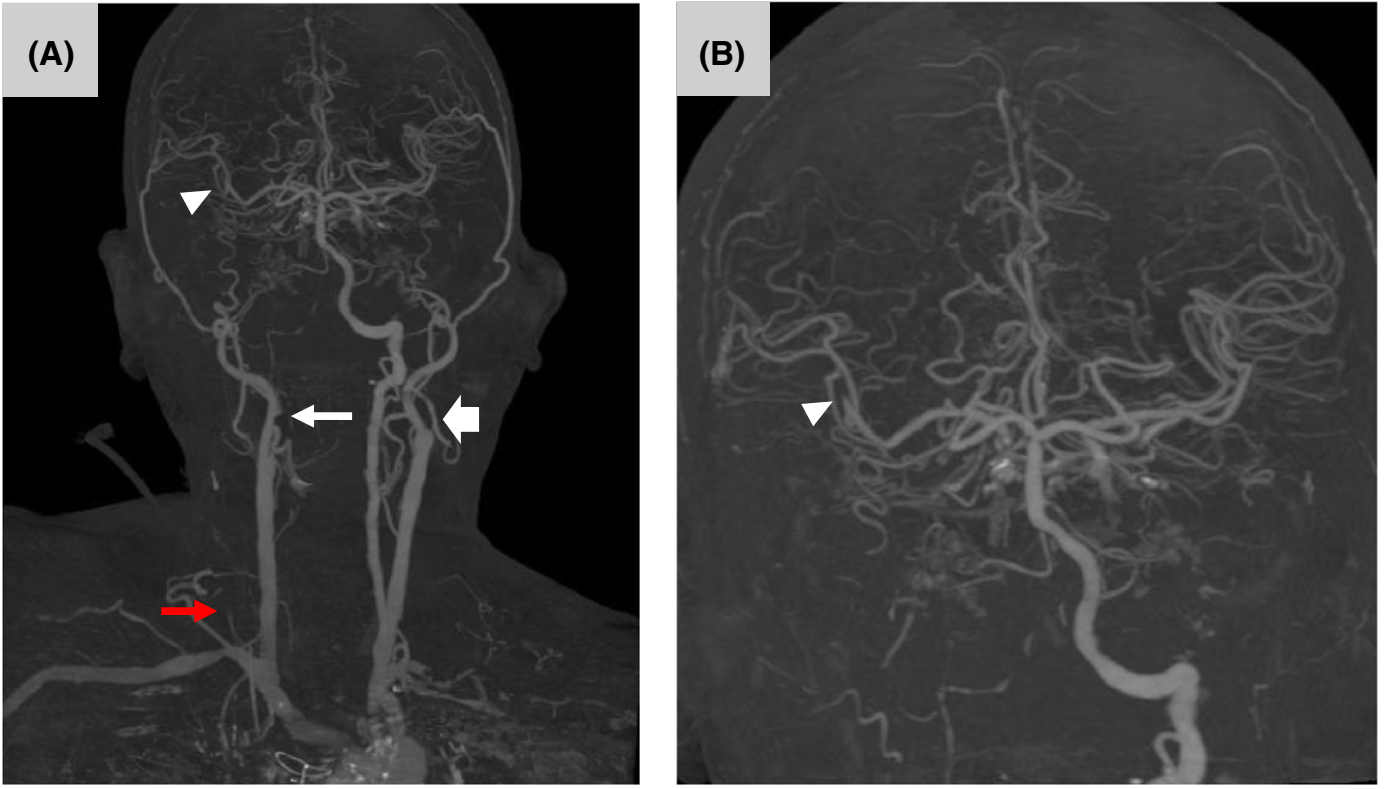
Three‐dimensional reconstructed images of CT angiography of the neck and brain showed occlusion of the origin of the right ICA (A, white arrow), the origin of the left ICA (A, wide arrow), the origin of the right vertebral artery (A, red arrow), and the M2 segment of the middle cerebral artery (A, B, arrowhead)
